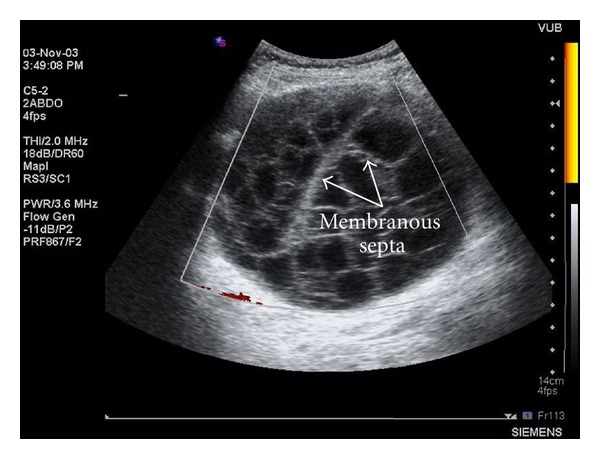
Abdominal ultrasonography (US) showing a well-defined large cystic mass in the right hypochondrium with several membranous septa (arrow).
